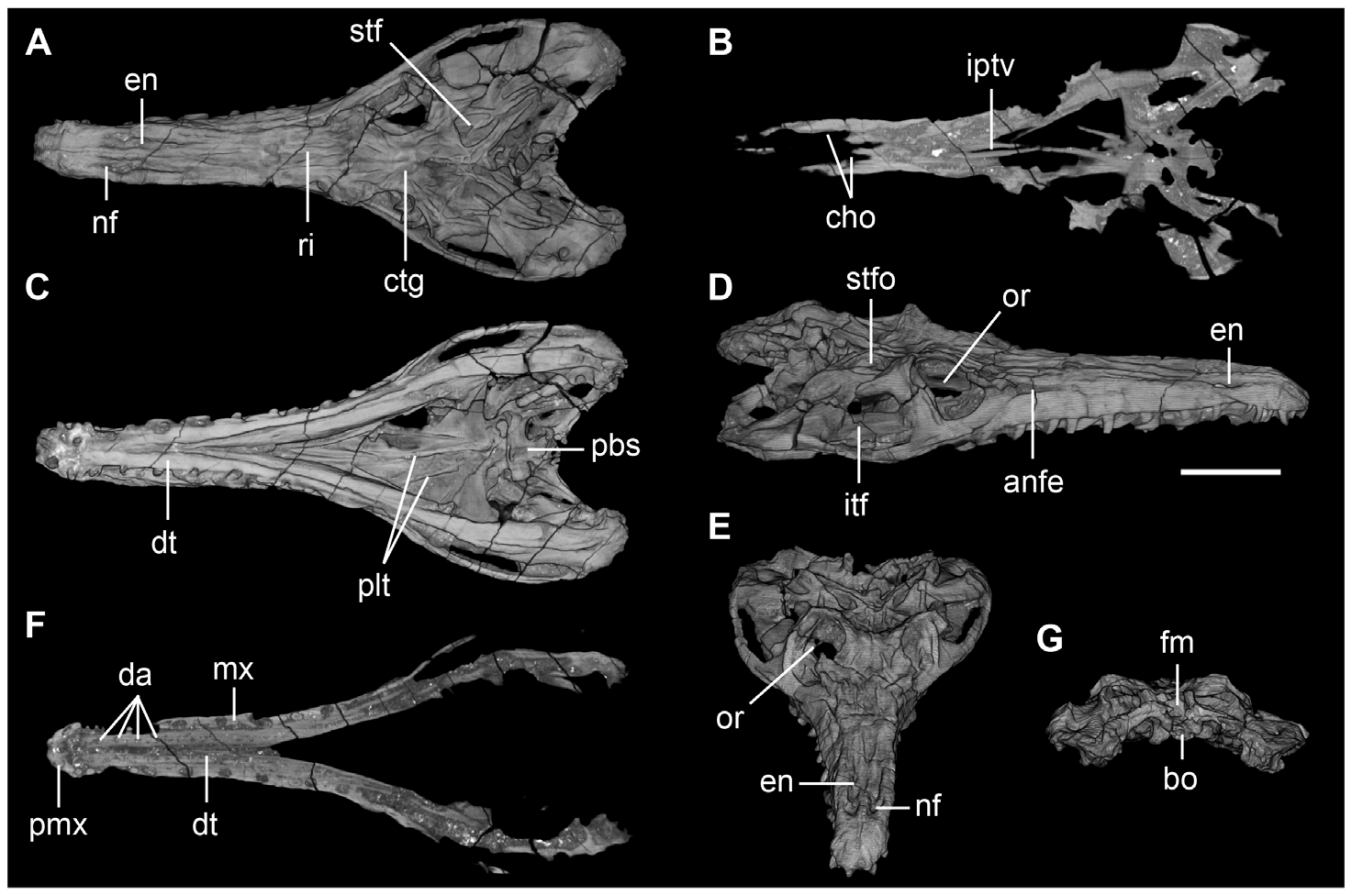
CT-scan slices and 3D reconstruction of the skull of *Pseudochampsa ischigualastensis* (PVSJ 567) in (A) dorsal view, (B) slice at level of the palate, (C) ventral, (D) dorsolateral, (E) and anterodorsal view, (F) slice at level of the dentary alveoli, and (G) occipital view. Abbreviations: anfe, antorbital fenestra; bo, basioccipital; cho, choanae; ctg, centre of growth; da, dentary alveoli; dt, dentary; en, external naris; iptv, interpterygoid vacuity; itf, infratemporal fenestra; fm, foramen magnum; mx, maxilla; nf, narial fossa; or, orbit; pbs, parabasisphenoid; pmx, premaxilla; plt, palate teeth; ri, ridge; stf, supratemporal fenestra, stfo, supratemporal fossa. Scale bar equals 5 cm.
